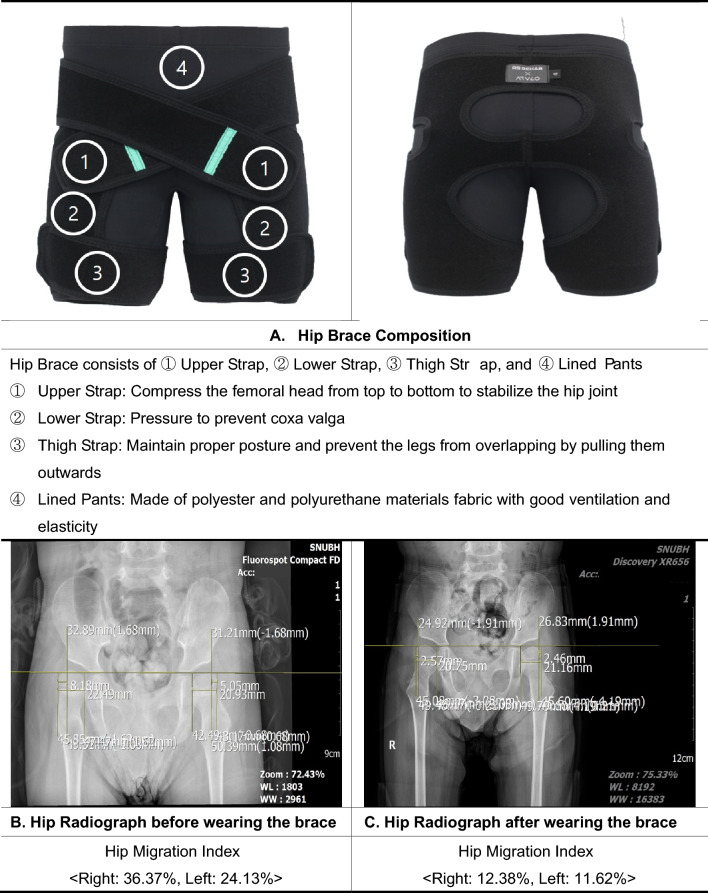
Hip brace composition and example radiographs of a patient.
